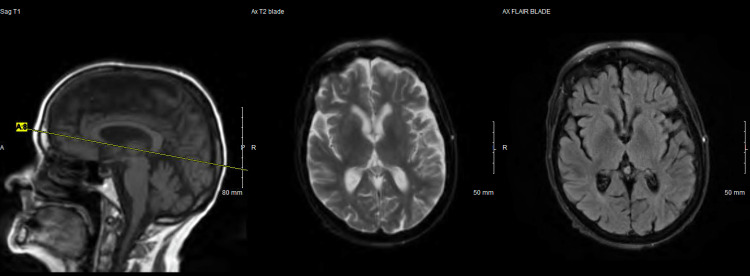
Magnetic resonance imaging with/without contrast of the head 2022 (1)
